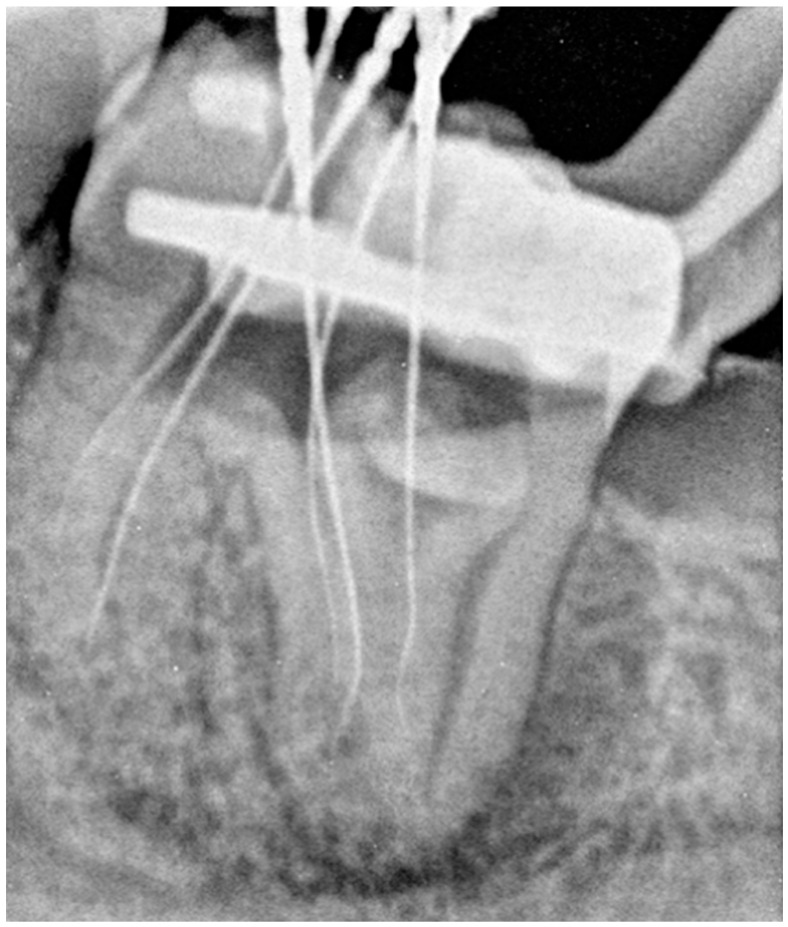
Tooth 3.7—Radiograph with five C-pilots size 10 (the first appointment).
